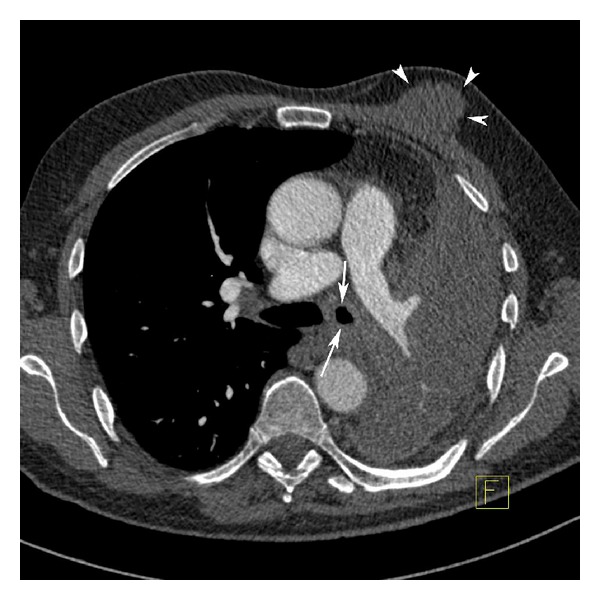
Thoracic CT shows massive pleural effusion and the left main bronchial obliteration (arrows) in the left lung. CT also shows the solid mass (arrowheads) in the left breast.
